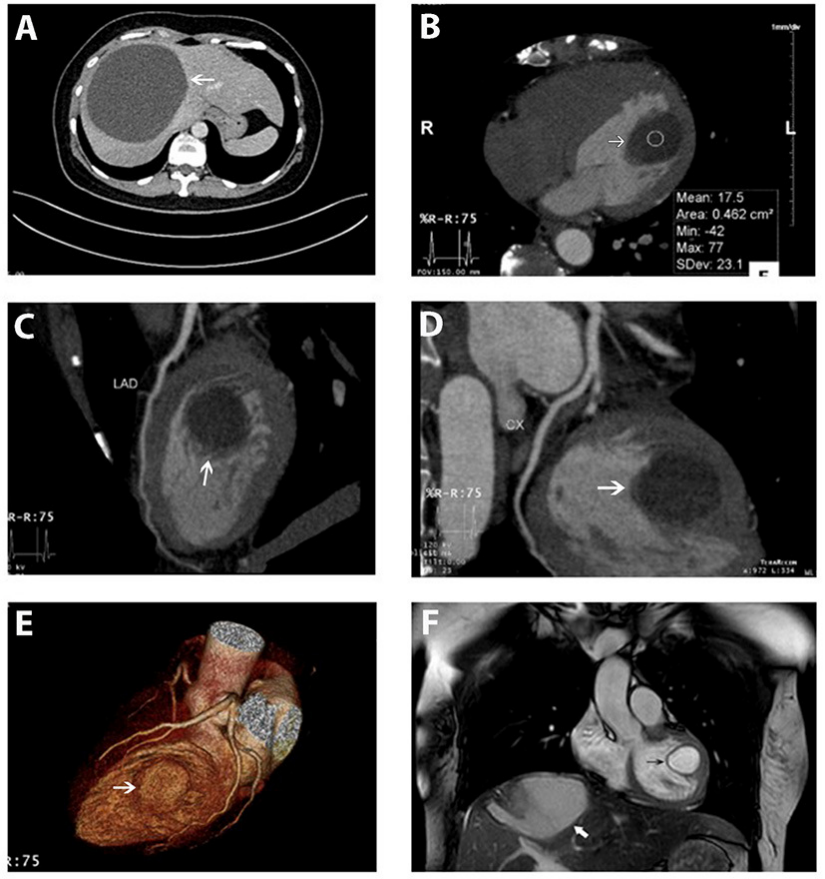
Hepatic cyst and the cardiac cyst on CT and on MRI. (A) A giant hepatic cyst was seen on the abdominal CT scanner (white arrow). (B–E) An encapsulated, rounded, and fluid-attenuated non-calcified structure along the LV lateral wall (white arrow) and its spatial relationship with coronary arteries (left anterior descending, circumflex) on MDCT. (F) Cardiac cyst (black arrow) and the hepatic cyst (white arrow) were simultaneously depicted on MRI. The cardiac cyst was a single intramuscular cyst located at the anterolateral papillary muscle attached to the LV lateral free wall, protruding into the LV cavity, not enhanced by the gadolinium. Myocardial perfusion and enhancement were normal.
